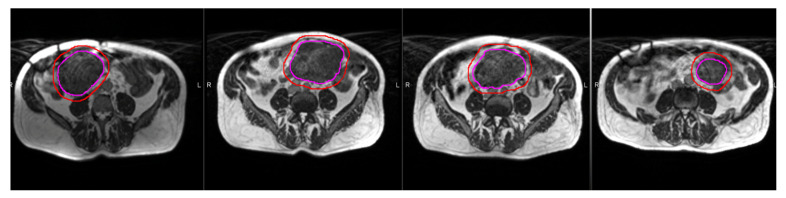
Treatment response assessment on serial MR images during a course of radiotherapy, with images moving chronologically from left to right. Note the visual reduction in gross tumor volume (pink) and overall reduction subsequently expanded target volume (red), as well as the freely mobile target in the abdominal cavity across multiple weeks.
